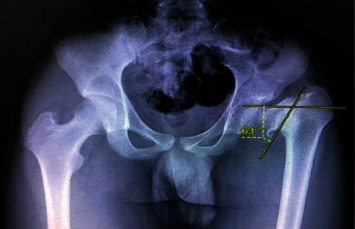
AP radiograph of the pelvis showing pseudoarthrosis of femur neck fracture with significant varus deformity: neck-shaft angle, 75 degree; Pauwels type 3, 63 degree.
